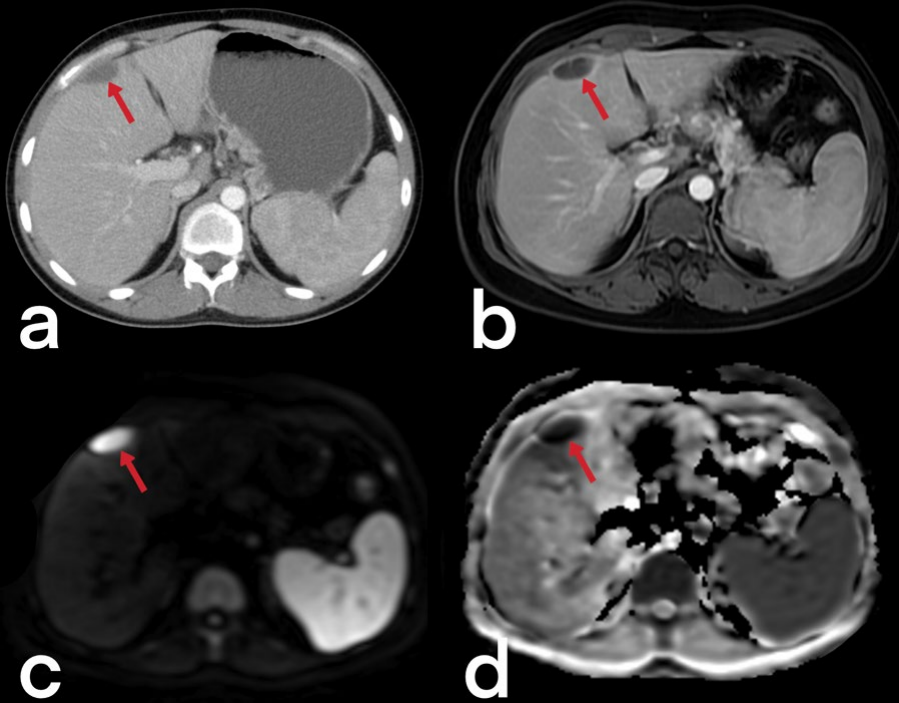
Abdominal CT scan and MR images of the same patient (in Fig. 27). Contrast-enhanced CT (a) and T1 weighted contrast-enhanced MR images (b) show a small abscess formation with rim-like enhancement in the anterior subcapsular region of the liver. Diffusion-weighted images (DWI) (c) and apparent diffusion coefficient (ADC) map (d) reveal diffusion restriction consistent with an abscess
